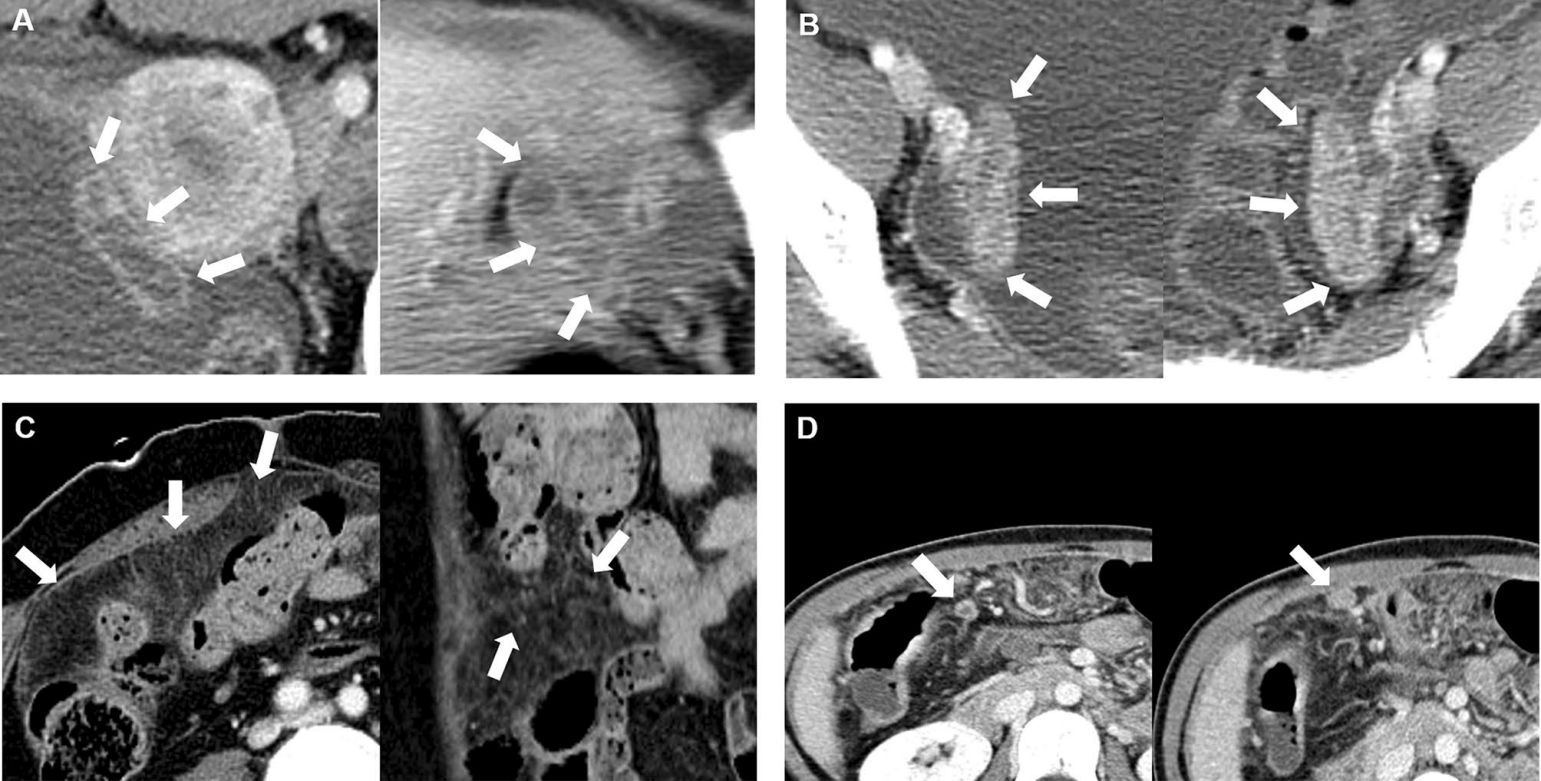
Representative cases of CT imaging features. (A) Hydrosalpinx, (B) fallopian tube enhancement, (C) peritoneal micronodule, and (D) peritoneal macronodule.
